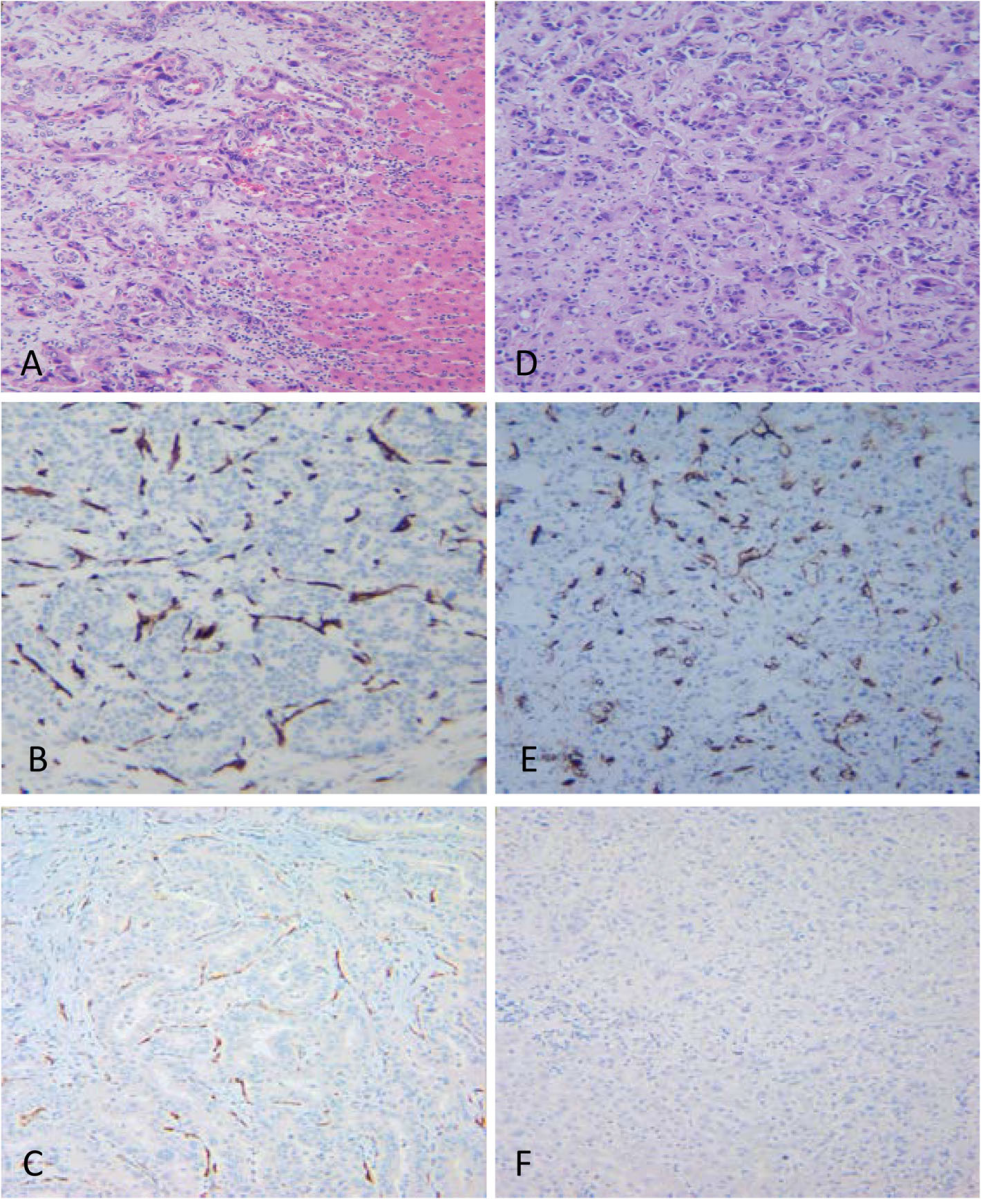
Primary cholangiocarcinoma and metastatic pancreatic ductal adenocarcinoma. a. Primary cholangiocarcinoma (HE, 100x); b. CD34 expression in cholangiocarcinoma (CD34, 200x); c. PSMA peritumoral/vascular expression in cholangiocarcinoma (PSMA, 200x). d. Metastatic pancreatic ductal adenocarcinoma in liver (HE, 100x) e. CD34 expression in pancreatic ductal adenocarcinoma (CD34, 200x); f. PSMA is negative in metastatic pancreatic ductal adenocarcinoma (PSMA, 200x)
